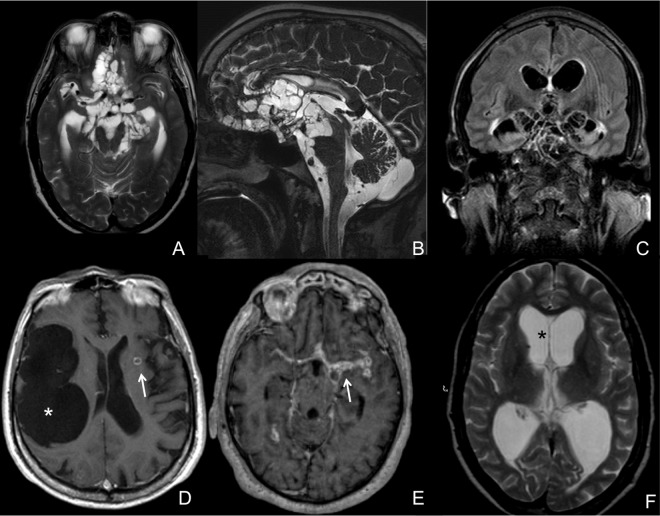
Different presentation patterns of extraparenchymal neurocysticercosis as revealed by brain MRI. Grape-like lesions in the basal cisterns are evident on T2 axial (A) and sagittal (B) views of a patient’s brain. C, An axial fluid-attenuated inversion recovery (FLAIR) coronal view of the same patient showing lesions in the Sylvian and interhemispheric fissures as well as basal cisterns. D, A giant cyst (*) in the right Sylvian fissure and a parenchymal enhancing cyst (arrow). E, A T1 axial view showing contrast enhancing in the Sylvian fissures (arrow), mainly on the left, which is associated with intense inflammatory reactions due to degenerating cysts. F. Ventricle enlargement (*) in a patient with neurocysticercosis-associated hydrocephalus.
